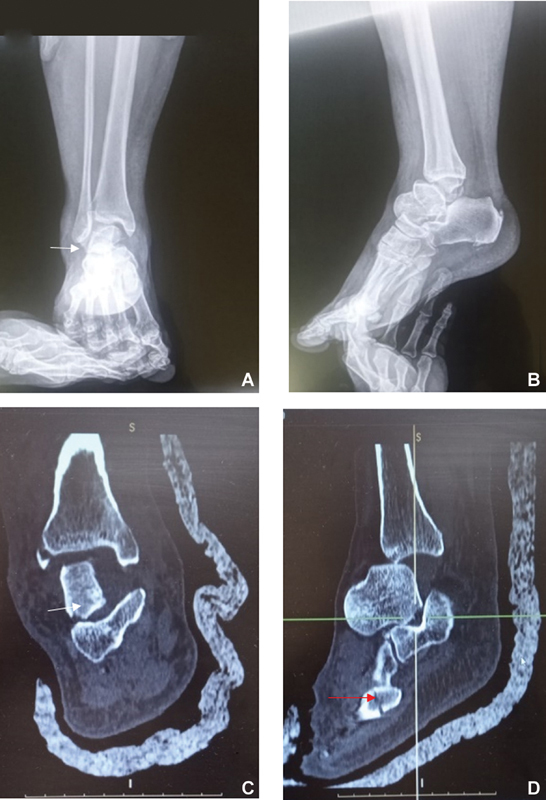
Plain radiographs and computed tomography scans showing total talar dislocation. (
